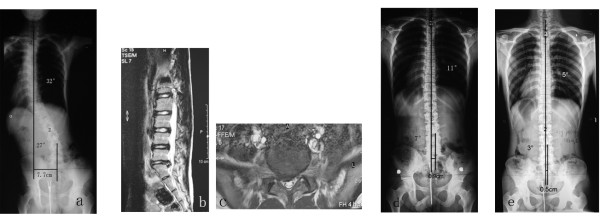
18.4-years-old-boy with L5-S1 disc herniation and scoliosis (patient No. 16). (a) Preoperative AP radiograph showed 32° left thoracic curve (T2-L2) and 27° right lumbosacral curve (L2-S1), the trunk shift was 7.7 cm. (b, c) MRI revealed L5-S1 disc space narrowing and paracentral disc protrusion with impingement of the right S1 nerve root. (d) Immediately after surgery, radiograph showed 11° thoracic curve and 7° lumbosacral curve with the trunk shift as 0.9 cm. (e) Anteroposterior radiograph taken 2.5 years after surgery showed 5° thoracic curve and 3° lumbosacral curve, the trunk shift was 0.5 cm, considering as being in normal condition.
